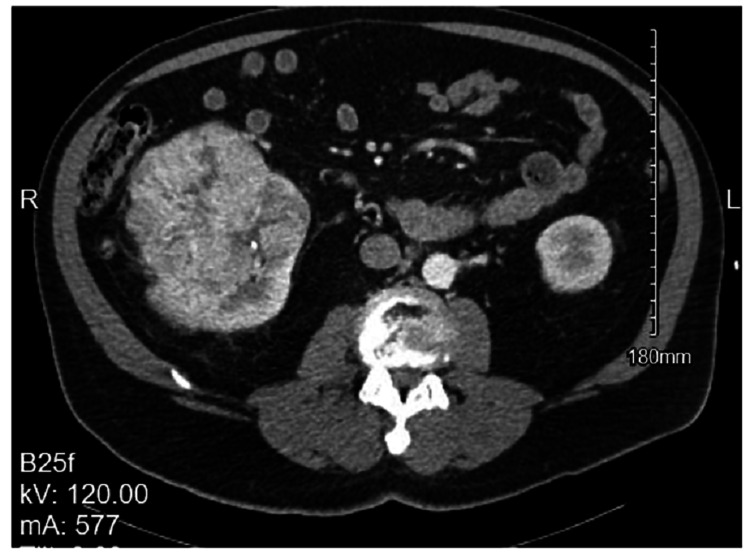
CT abdomen and pelvis with contrast showing right-sided renal cell carcinoma
